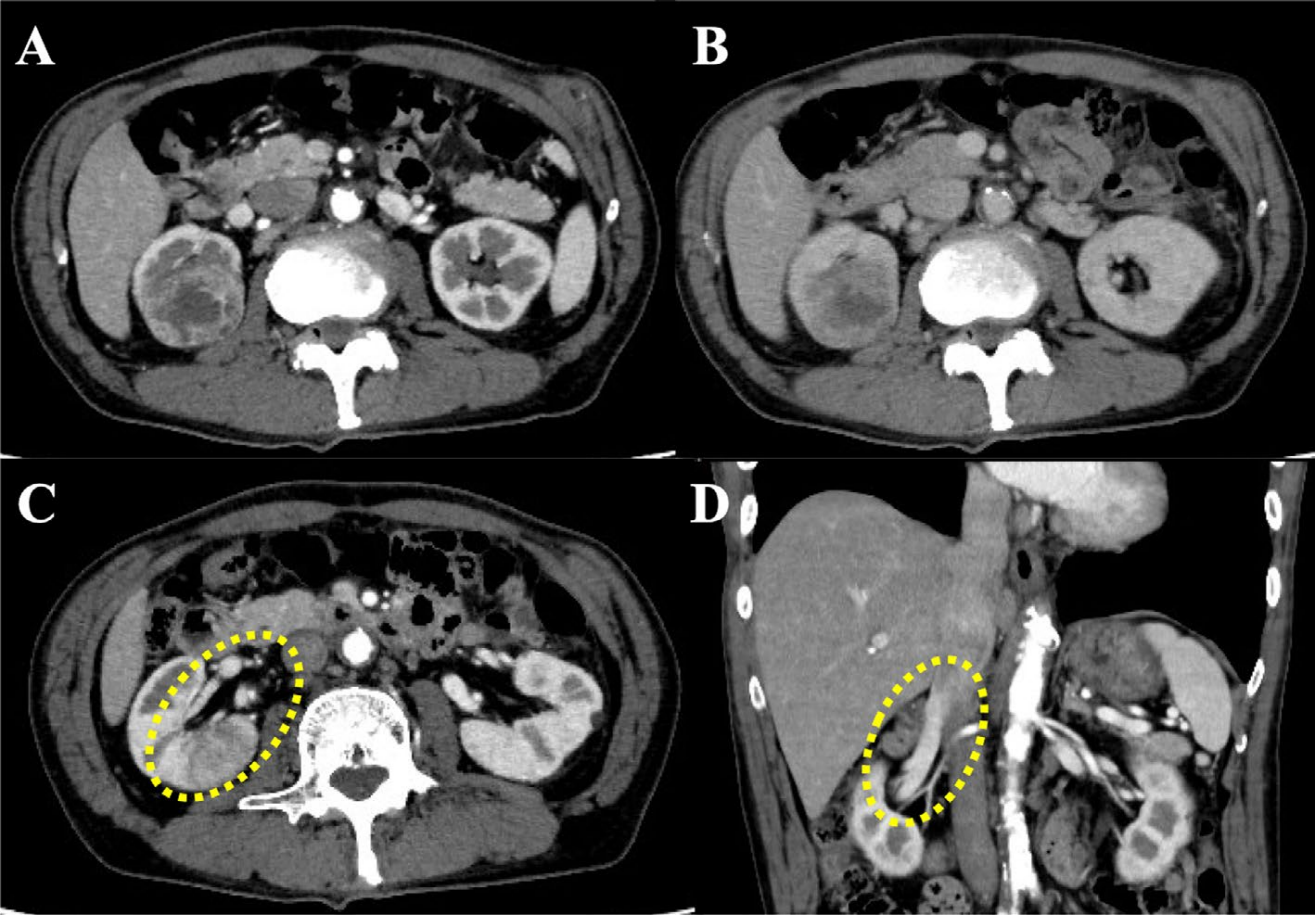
Computed tomography (CT) findings. (A: arterial phase, B: equilibrium phase) CT showed a tumor in the upper pole of the right kidney. (C) There was no tumor thrombus in the outflow vein (yellow dotted circle). (D) There was no tumor thrombus in the renal vein (yellow dotted circle).
